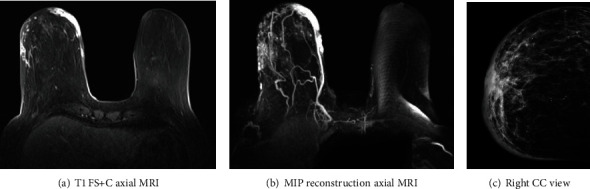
(a–c) This is a different case. An 80-year-old patient with remote history of right breast lumpectomy and radiation treatment due to breast malignancy. Patient presented with similar clinical symptoms of skin changes and discoloration. Axial T1 with fat suppression (FS), postcontrast injection (+C) MRI (Figure 6(a)), and MIP reconstruction (Figure 6(b)) demonstrating marked skin thickening and enhancement. Corresponding right CC mammographic view (Figure 6(c)) demonstrating marked skin thickening with no discreet mass within the breast.
